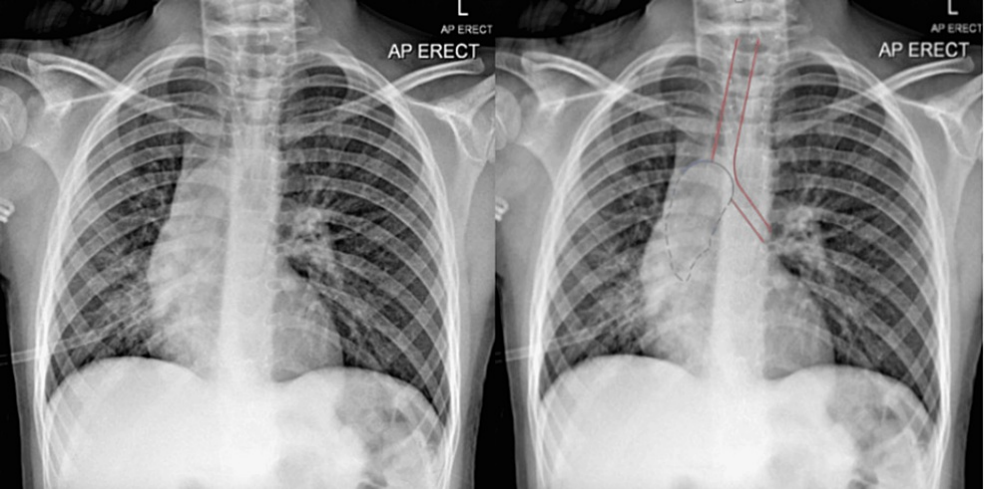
Left side: Presenting chest x-ray (CXR). Right side: CXR annotated; Solid red line: trachea and left main bronchus. Solid blue line: tumour projecting into distal trachea at the carina. Interrupted blue line: distal atelectasis and airways plugging behind the endobronchial tumour
